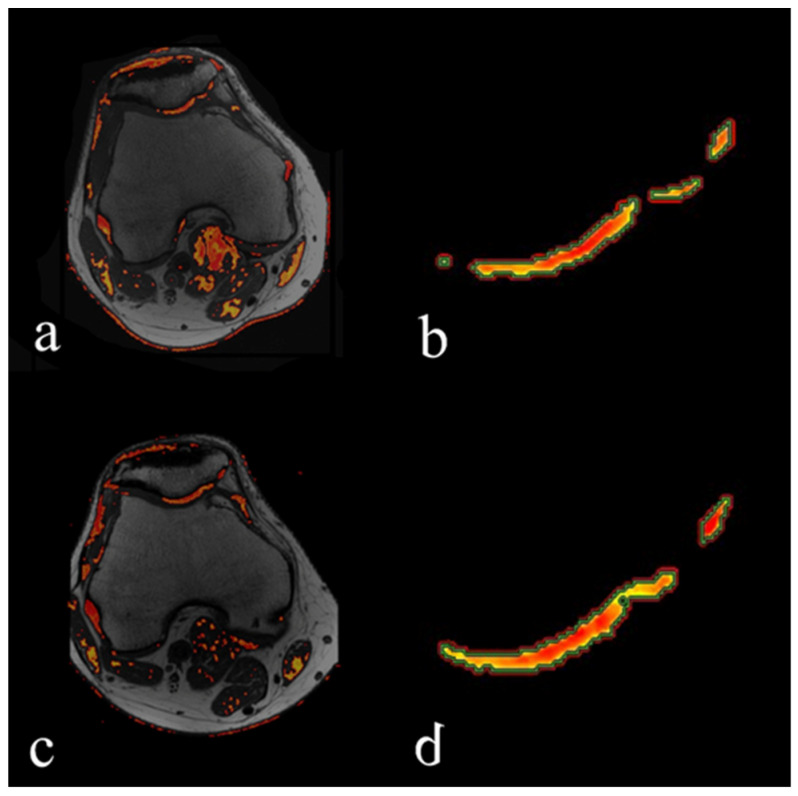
MR imaging follow-up in a 37-year-old patient at baseline (M0, (a,b)) and at 12 months (M12, (c,d)). The volume of healthy cartilage was estimated at 352 mm^3^ at M0 on T2 mapping (a,b) and at 731 mm^3^ at M12 (c,d). Images (b,d) are magnified views of the patellofemoral junction in (a,c), respectively.
